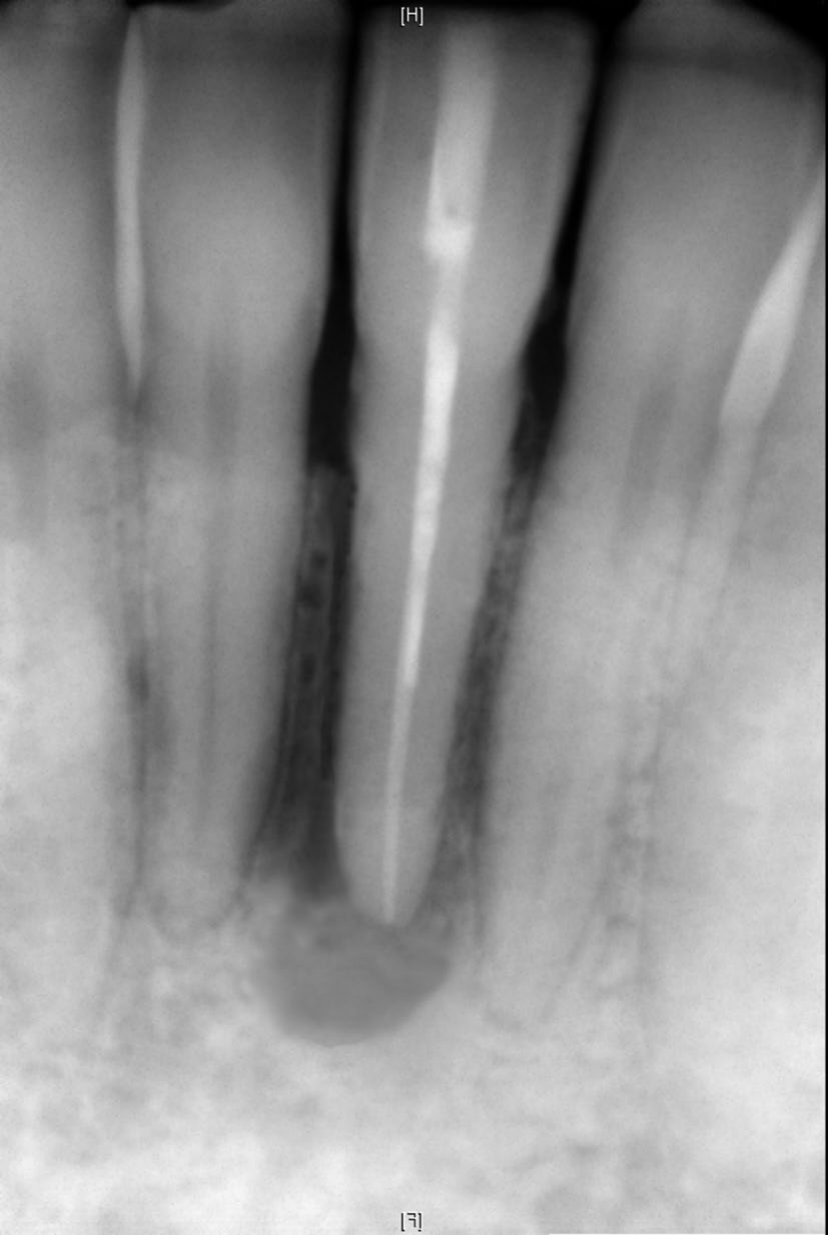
Intraoral radiograph of the anterior mandibular region. Tooth 31 shows a typical lateral J‐halo lesion due to a vertical root fracture.
